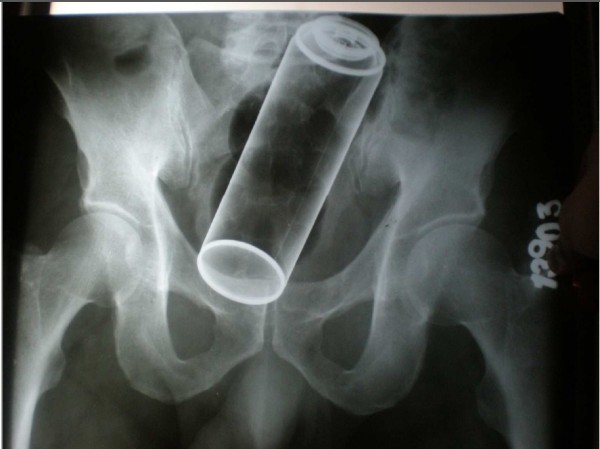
Rectal ımpulse body spray can on abdominal plain film.
